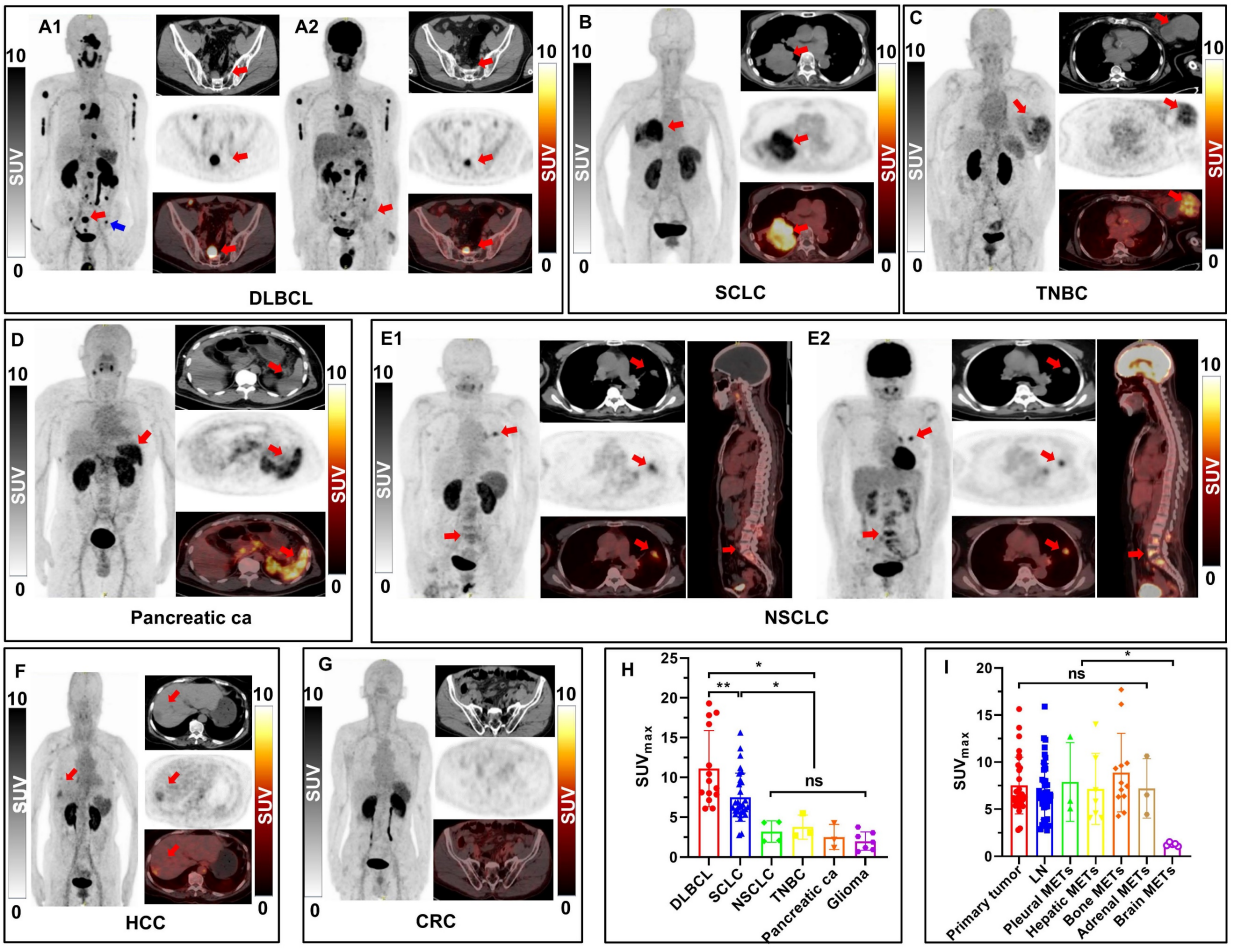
[^18^F]AlF-NOTA-QHY-04 PET/CT scanning of patients with hematologic malignancies or different solid tumors. (A-G) For each case, maximum-intensity projections (MIP) are presented on the left, with corresponding transaxial CT (top), PET (middle), and fused PET/CT (down) images shown on the right. A2 and E2 are [^18^F]FDG PET/CT images, the others are [^18^F]AlF-NOTA-QHY-04 PET/CT images. Red arrows indicate CXCR4-positive tumor lesions; the blue arrow indicates the inguinal lymph node. (H) SUV_max_ of [^18^F]AlF-NOTA-QHY-04 in 6 types of tumor patients. (I) Comparison of SUV_max_ values in primary tumors and metastases of 30 SCLC patients. ns, not statistically significant. P* < 0.05, *P* < 0.01.
